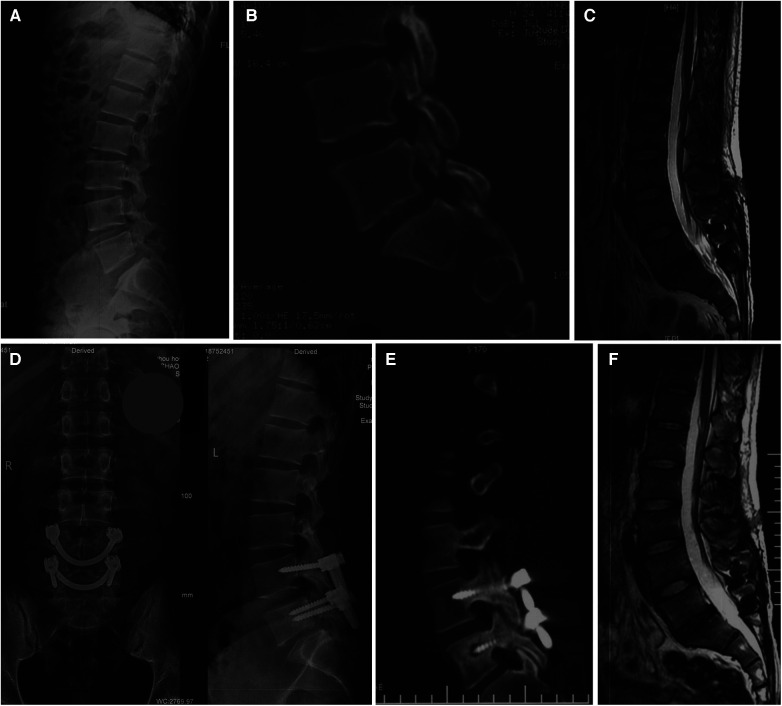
Preoperative and postoperative images obtained in a 26-year-old male diagnosed with double-level lumbar spondylolysis. (A–C) Spondylolysis at L4 and L5. (D–F) Postoperative radiographs.
